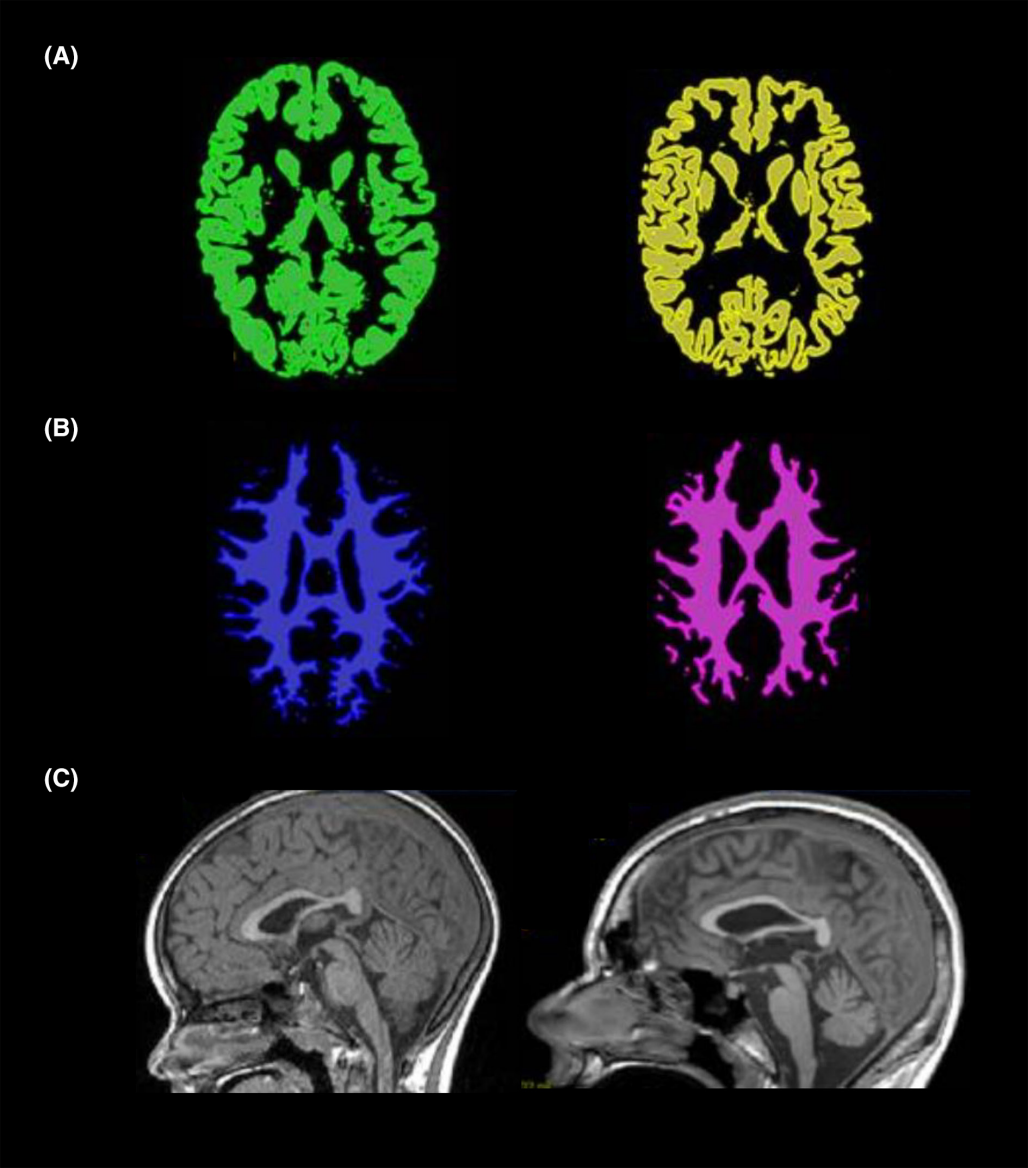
MRI of patient #1 at age 6.8 years prior to VPA initiation (left column) and at age 11.1 years on VPA treatment for 23 months (right column). Loss of cerebral gray matter (A), white matter (B) and cerebellar atrophy (C) are seen on second MRI compared to first one.
